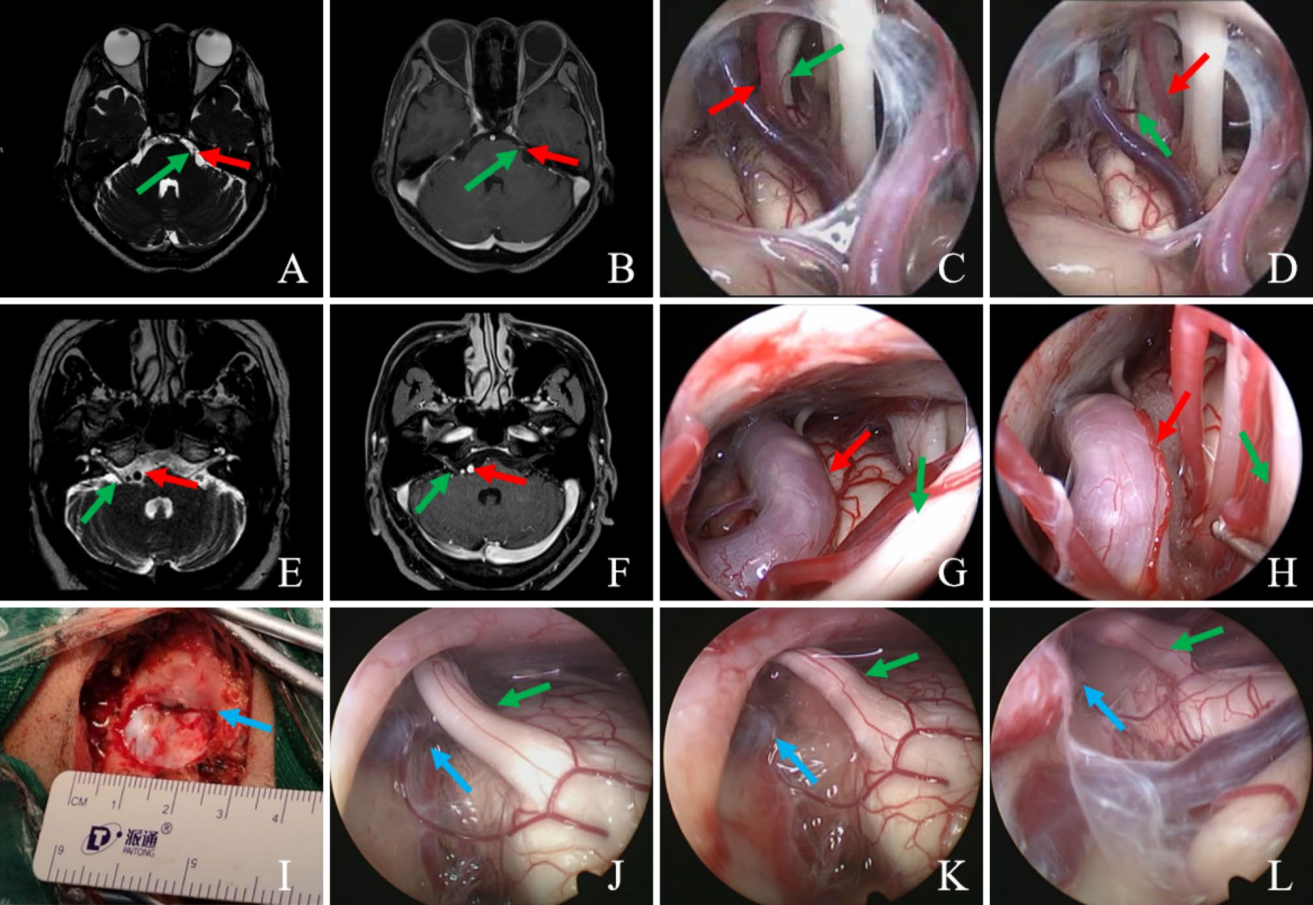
A-D, A case of TN. (A-B): Images A and B show the patient’s preoperative 3D FIESTA MRI and 3D-TOF MRA imaging, respectively, with the green arrow pointing to the trigeminal REZ and the red arrow pointing to the responsible vessel, which forms a compression in the REZ of the TGN; (C-D): Pictures C and D show that the responsible artery (indicated by the red arrow) forms a neurovascular compression from the trigeminal nerve (indicated by the green arrow) REZ to Meckel’s bursa throughout, and that the neurovascular decompression is adequate and definitive with the aid of the endoscope with a Teflon cotton placed under direct vision. E-H, A case of HFS. (E-F): Images E and F show the patient’s preoperative 3D FIESTA MRI and 3D-TOF MRA imaging, respectively. The dilated displaced basilar artery (indicated by the red arrow) squeezes the ipsilateral nerve (indicated by the green arrow); (G-H): Thick basilar artery (indicated by the red arrow) squeezing the REZ of the FN (indicated by the red arrow), with high tension between them and the brainstem. Intraoperatively, the basilar artery is cushioned away from the FN using “pre-placed” technique and “set up bridge” technique to achieve adequate decompression. I, Surgical incision and bone window. The surgical design creates an elliptical microbone window of approximately 2.5 cm in diameter, revealing the junction of the transverse and sigmoid sinuses (indicated by the blue arrow). J-L, A case of TN with venous-type compression. Intraoperatively, the responsible vein (indicated by the blue arrow) was seen to form a compression beneath the TGN (indicated by the green arrow), which was adequately decompressed by the operator using “pre-placed” technique, and the decompression was checked by “diving” technique at the end of the procedure
